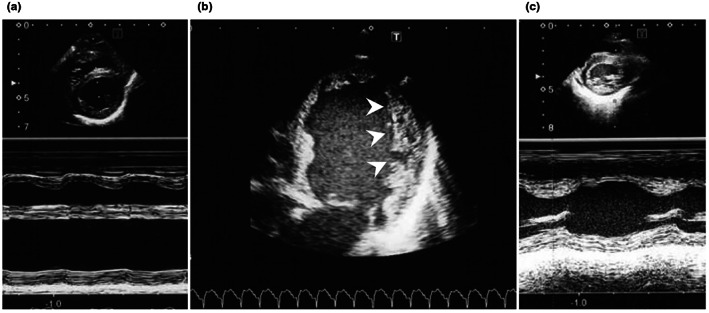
Echocardiographic findings. (a) M‐mode echocardiography from a parasternal left ventricular short‐axis view on admission. The left ventricular diameter was dilated (left ventricular end‐diastolic diameter was 150% of normal) and cardiac pump function was markedly reduced (20% of left ventricular ejection fraction). (b) B‐mode echocardiography from an apical four chamber view. Prominent left ventricular trabeculae and deep intertrabecular recesses (arrowheads) were found. The ratio between the noncompacted and compacted layers was calculated to be 2.0 at the lateral side of the left ventricular wall at the end‐diastolic phase, which meets the criteria for left ventricular noncompaction. (c) M‐mode echocardiography from a parasternal left ventricular short‐axis view 2 months after admission. The left ventricular diameter was reduced (left ventricular end‐diastolic diameter was 116% of normal) and cardiac pump function was improved (left ventricular ejection fraction 55%).
